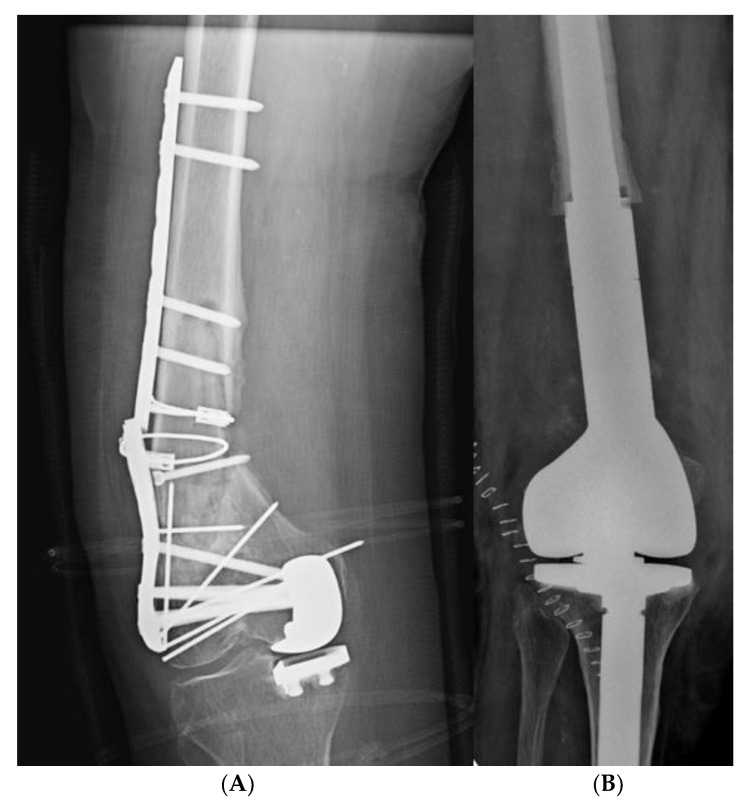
80-year-old patient with 33-A2 femur fracture. (A) Anteroposterior X-ray of the femur showing a PSA is present in the distal third of the femoral diaphysis, associated with mono-compartmental knee prothesis, broken plate, multiple Kirshner wires and metal cerclages. (B) Anteroposterior knee X-ray showing knee mega prosthesis implantation as revision surgery.
